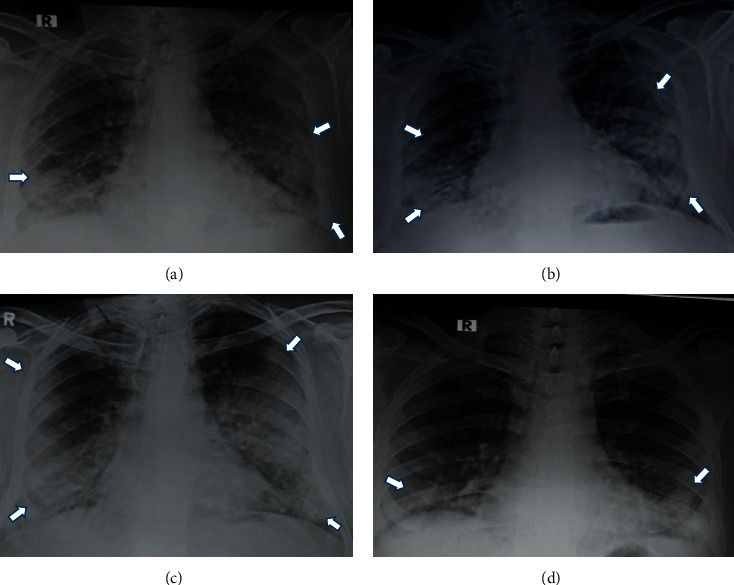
Evolution of X-ray findings in a COVID-19 patient. (a) Day 3: RALE score: 3. (b) Day 6: RALE score: 5. (c) Day 10: RALE score: 6. (d) Day 14: RALE score: 2 patient was discharged on day 21.
